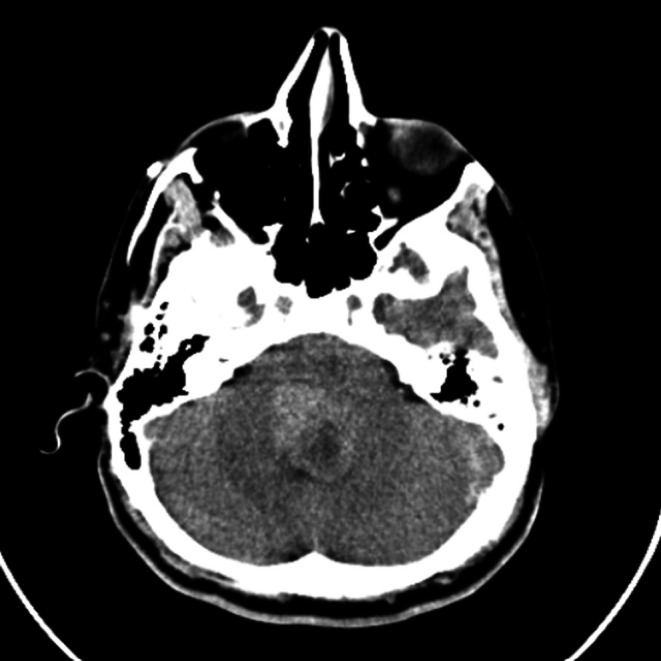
Axial plane of the patient's brain CT scan showing a heterogeneous hyperdense lesion located in the fourth ventricle.
